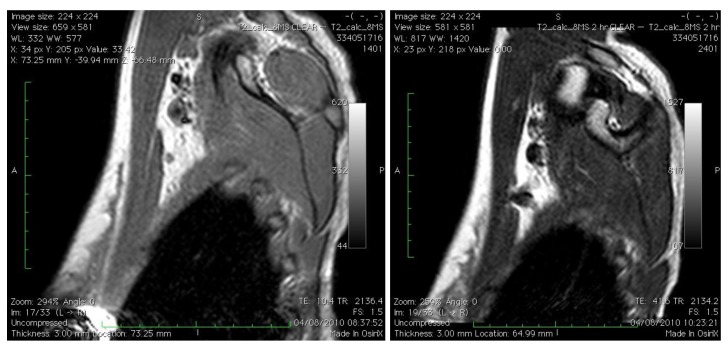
Left panel: right axilla pre-Endorem injection on MRI scans; right panel: SLN easily identified 120 minutes post-Endorem injection intradermally.
